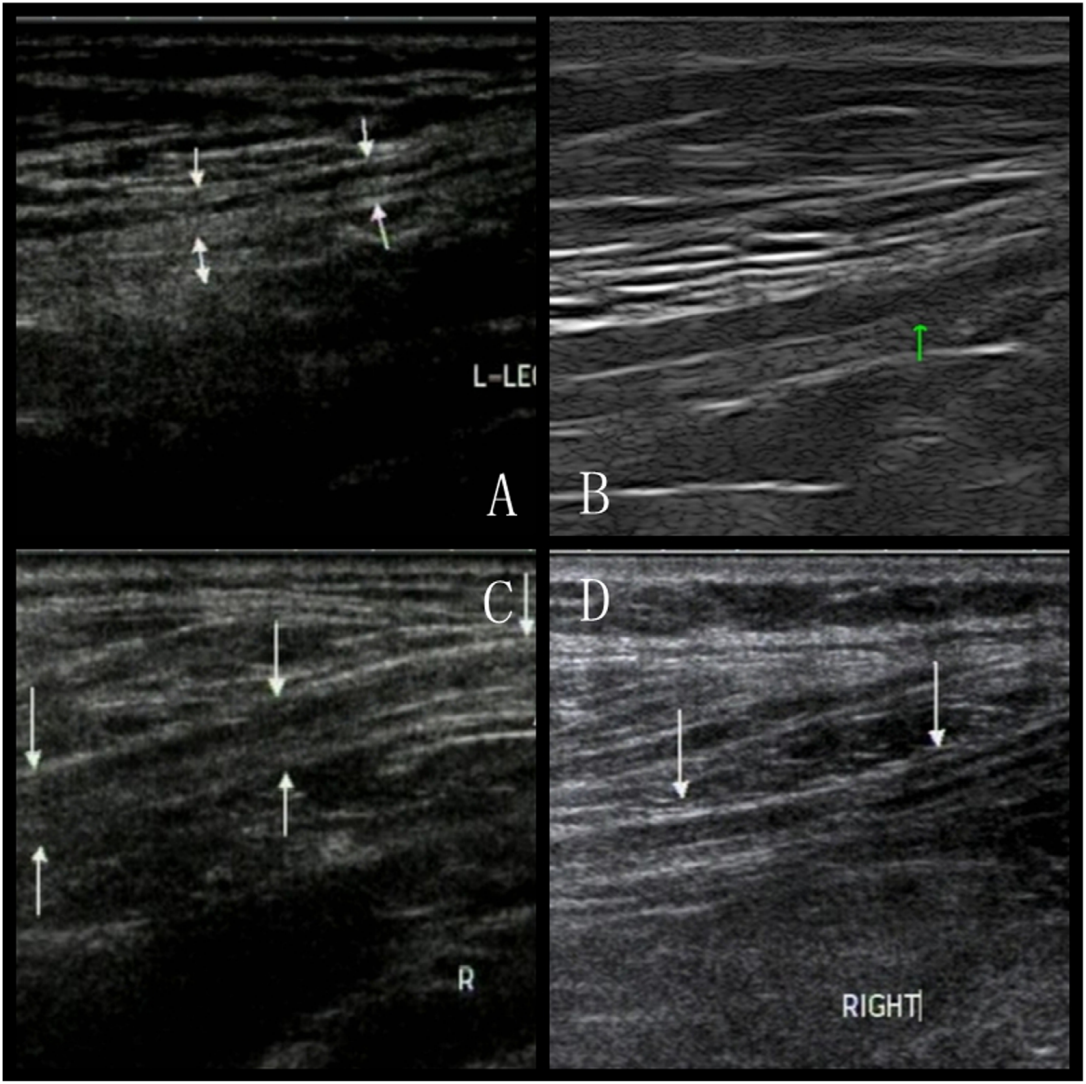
Longitudinal sonograms showing difference of morphological changes after surgical decompression between two patients with focal and diffuse painful DPN. A and B represent the preoperative (Da: 4.4 mm, Dt: 7.6 mm) and postoperative (Da: 2.9 mm, Dt: 6.3 mm) sonograms of the common peroneal nerve at the level of knee joint of patient with focal painful DPN. C and D represent the preoperative (Da: 4.2 mm, Dt: 7.0 mm) and postoperative (Da: 3.7 mm, Dt: 6.8 mm) sonograms of the common peroneal nerve at the level of knee joint of patient with diffuse painful DPN.
